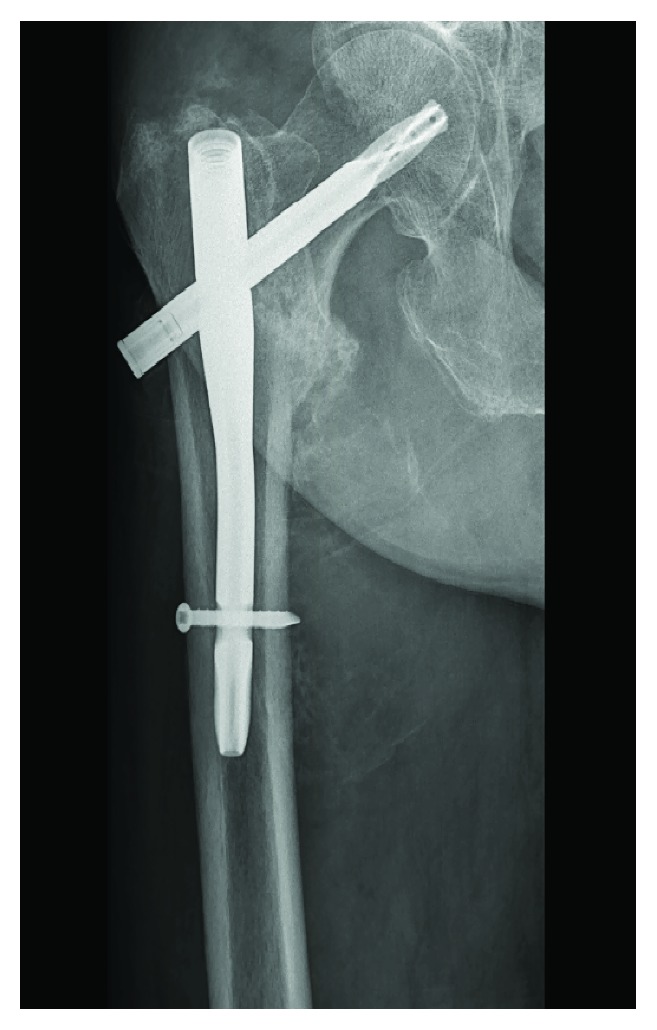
Plain X-ray of the right hip six weeks postoperatively showed a good position of the intramedullary nail.
